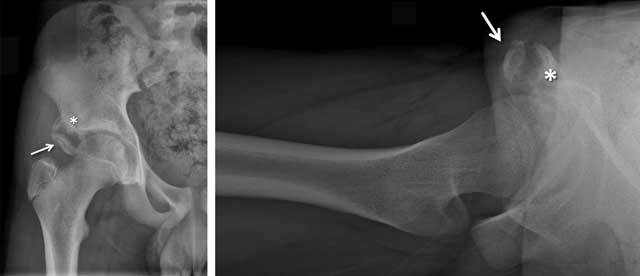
Anteroposterior and cross-table lateral radiograph of the right hip joint in a 15-year-old male soccer player who presented with a painful swelling and suspected neoplasia of the right hip region. Both the avulsed osseous fragment (arrow) and the osseous irregularities at the anterior inferior iliac spine (asterisk) are well visualized, as this was not a tumor but an avulsion injury at the origin of the rectus femoris muscle.
